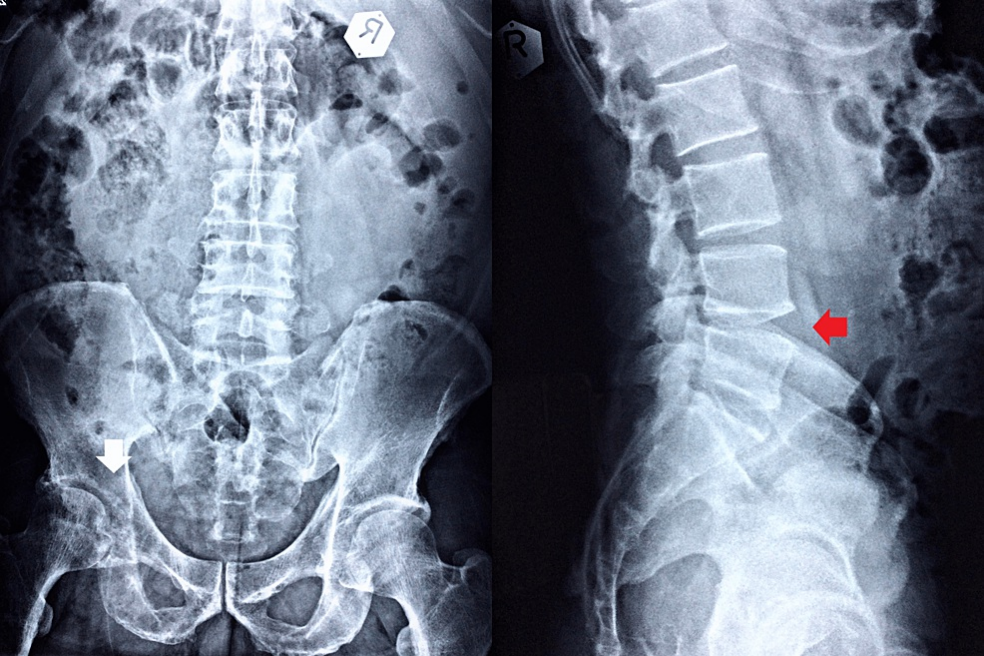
Lumbar and pelvic radiography The anteroposterior and lateral views appear to have reduced L4-5 disc space and a marginal anterior osteophyte in the lumbosacral spine (LS) vertebral body (red arrow). Multiple lucent foci are also found on the left pelvic rim, femoral head, and proximal body (white arrow).
